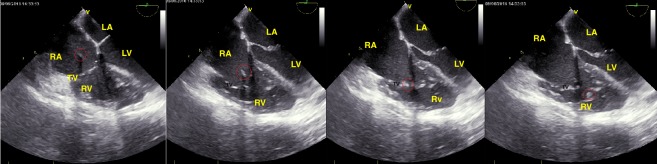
Serial echocardiographic pictures of catheter tip through the cardiac cycle The catheter tip is circled red. TV: tricuspid valve; RV: right ventricle; LV: left ventricle; TV: tricuspid valve; RA: right atrium; LA: left atrium
